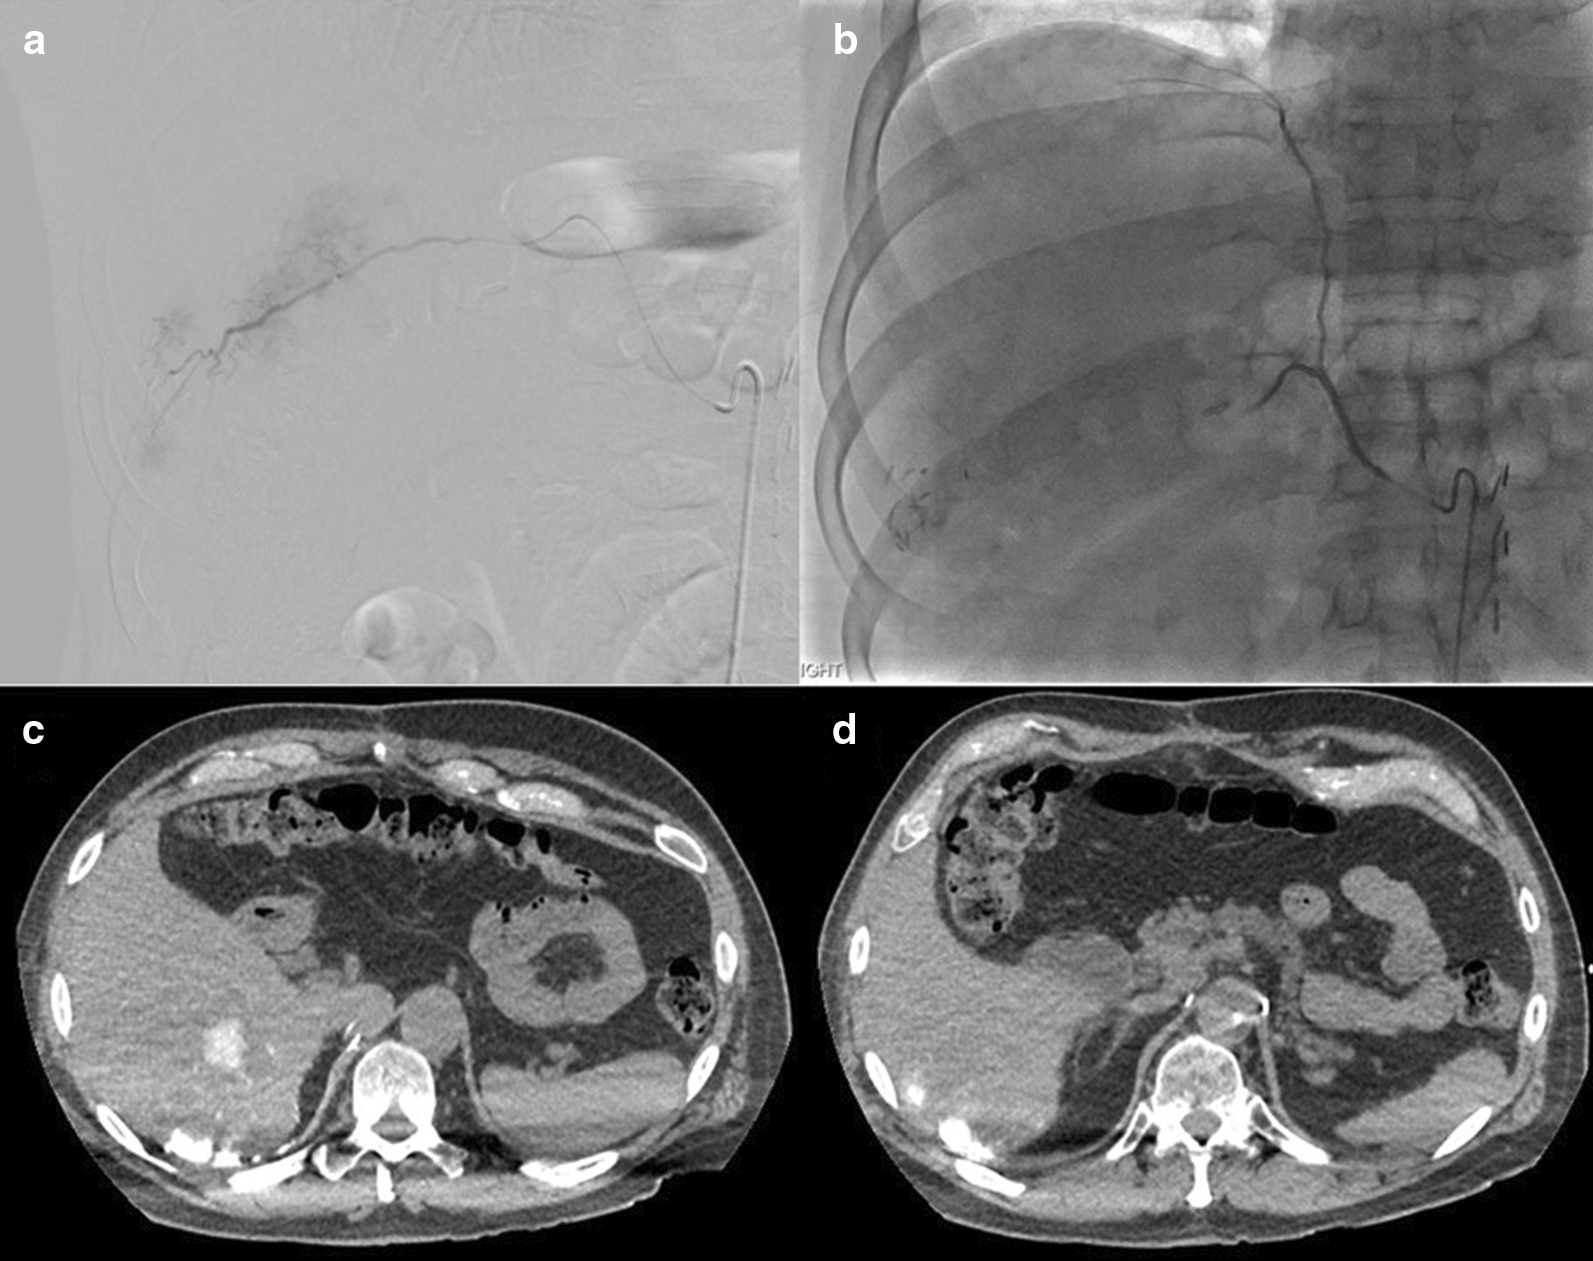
Right inferior phrenic artery. a The inferior branch of the right inferior phrenic artery was superselected, demonstrating tumor blush in the inferior right hepatic lobe. b Post-bland embolization of the inferior branch of the right inferior phrenic artery demonstrated stasis with persistent flow to the remaining branches of the right inferior phrenic artery. c, d Intraprocedural selective 3D contrast-enhanced CT angiogram of the inferior branch of the right inferior phrenic artery demonstrated enhancement of hepatic lesions along the posterior periphery of the right hepatic lobe
